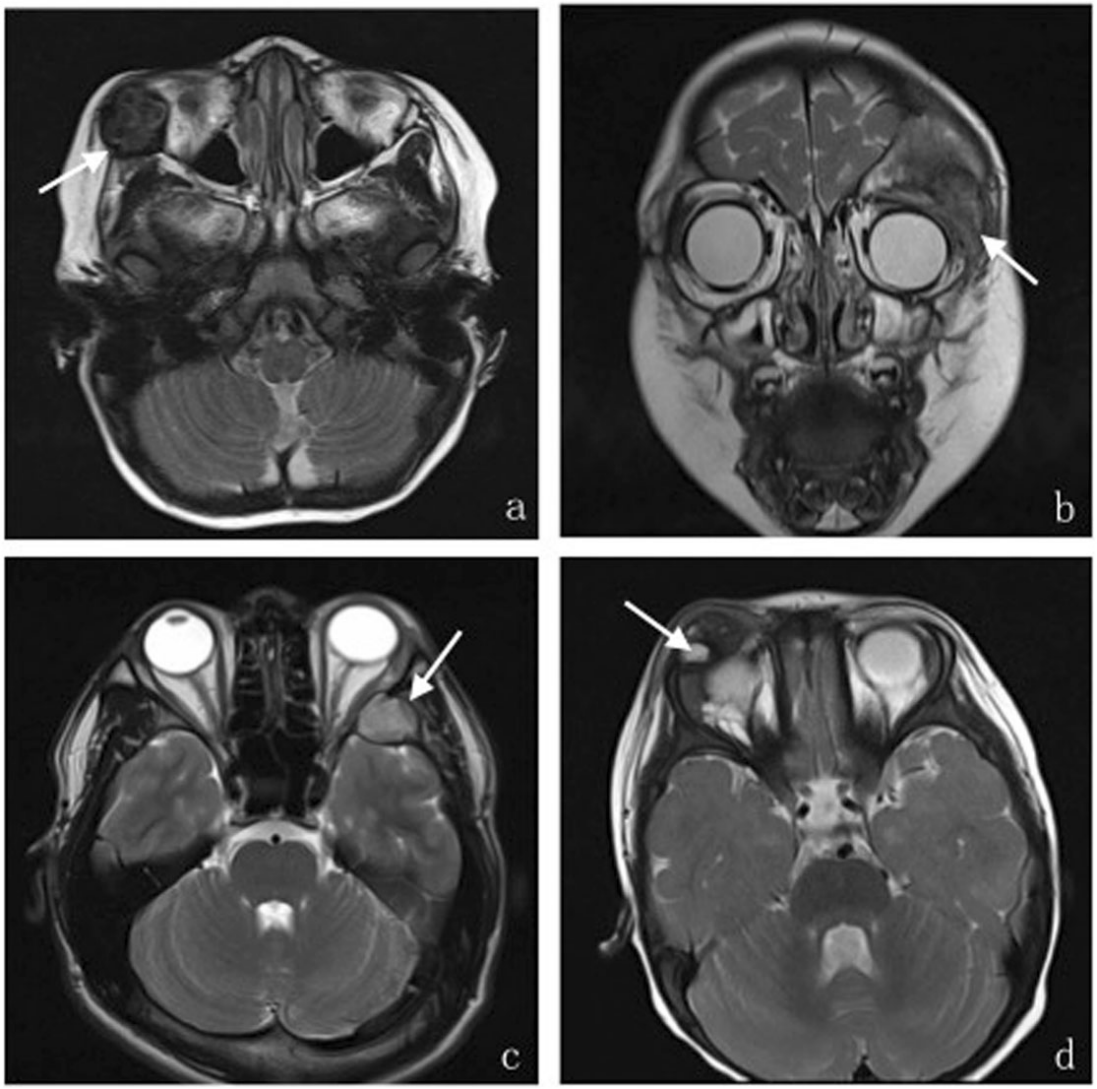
a–d. The different performances of orbital LCH lesions in T2WI:Axial (a) T2-weighted MR images show lesion located in the inferolateral right orbit with a clear border, and presenting hypointensity in a T2-weighted image with a low signal ring of the lesion edge;and coronal (b) T2-weighted MR images show lesion located in the right superolateral orbit with an irregular shape like a triangle and an unclear boundary, presenting hyper-hypointense mixed signals; Axial (c) T2WI show lesion located in the lateral wall of the left orbit presenting slightly hyperintense signals with a clear boundary. Axial (d) T2WI show lesion located in the superior wall of the right orbit presenting multiple cystic hyperintense signals near the edge of the lesion
